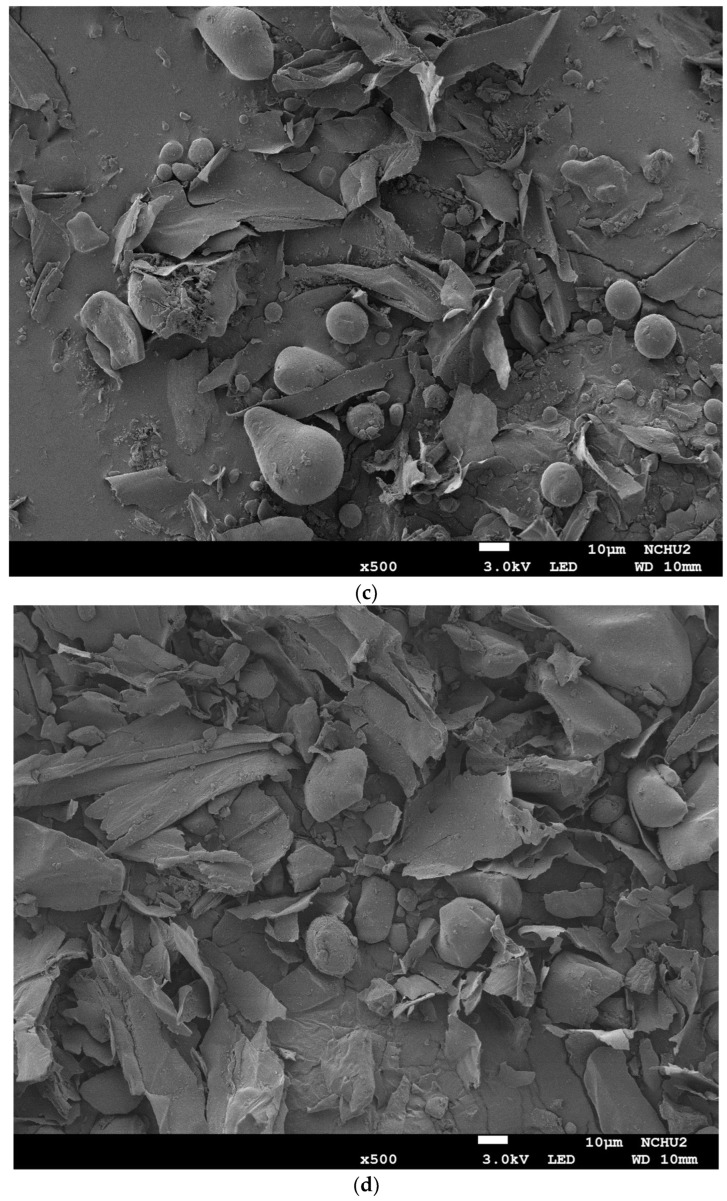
FESEM images (×500) of dried burdock root powder (DBR) and the residues of DBR via various extraction modes for the condition of 120 min of extraction and a 1/30 (g/mL-solvent) solid-to-liquid ratio at 30 °C using 95% ethanol as the solvent: (a) DBR; (b) residue of DBR by using shaking at 120 rpm (S120); (c) residue of DBR by using 40 kHz/300 W of ultrasound (U40); (d) residue of DBR by using 120 kHz/300 W of ultrasound (U120).
